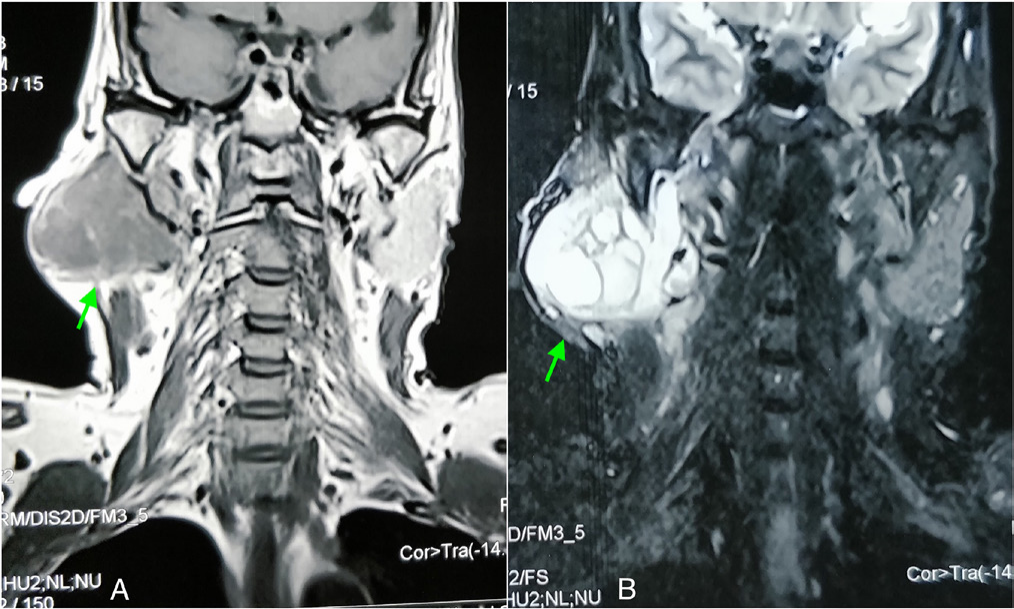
T1 (A) and T2 fat sat (B) coronal MRI images shows a lobulated well-circumscribed mass lesion involving both superficial and deep lobe of right parotid gland. The lesion shows hypointense signal on T1 and bright signal on T2.
